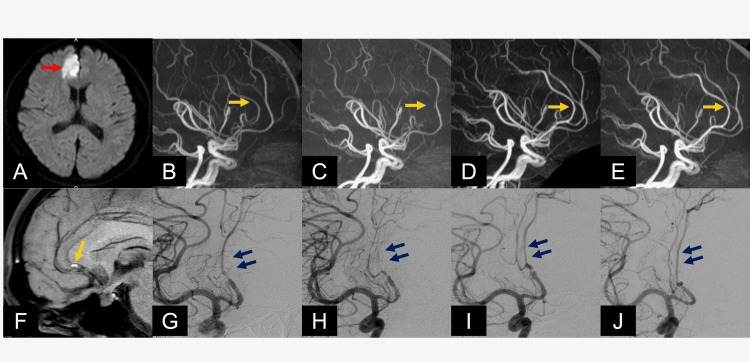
Case 1's radiological images A: An MRI at admission revealed acute cerebral infarction in the right anterior cerebral artery (ACA) territory (red arrow). The magnetic resonance angiography (MRA) showed at admission (B), after two weeks (C), after three months (D), and after one year (E) changes in findings of stenosis of ACD over time (orange arrow). F: Motion-sensitized driven-equilibrium sequence (MSDE) showed high intensity at stenosis of ACA, which suspected intramural hematoma (IMH) (yellow arrow). Digital subtraction angiography (DSA) at admission (G), after two weeks (H), after three months (I), and after one year (J) revealed finally normalization of ACD (blue arrows).
